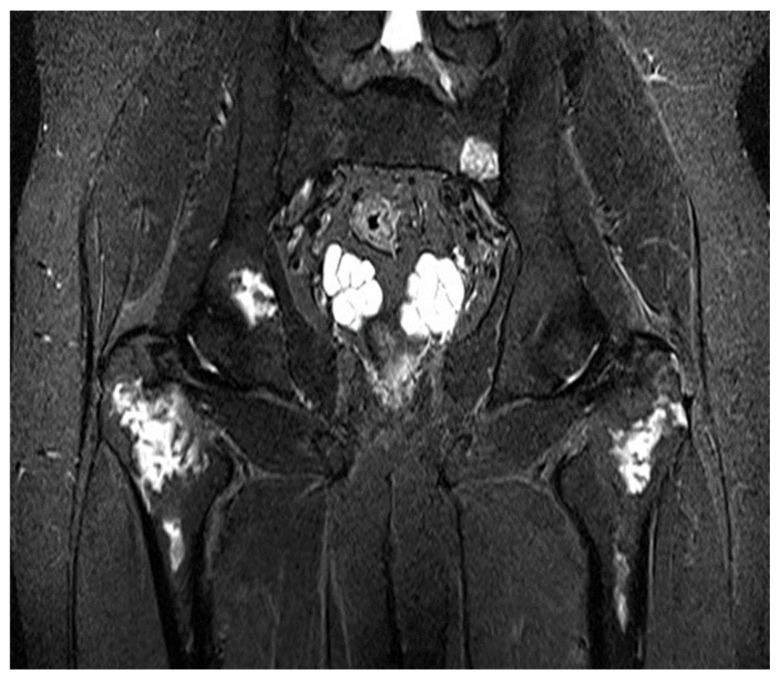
Coronal STIR MRI of a 27-year-old male patient with multiple areas of osteonecrosis and bilateral femoral head avascular necrosis.
